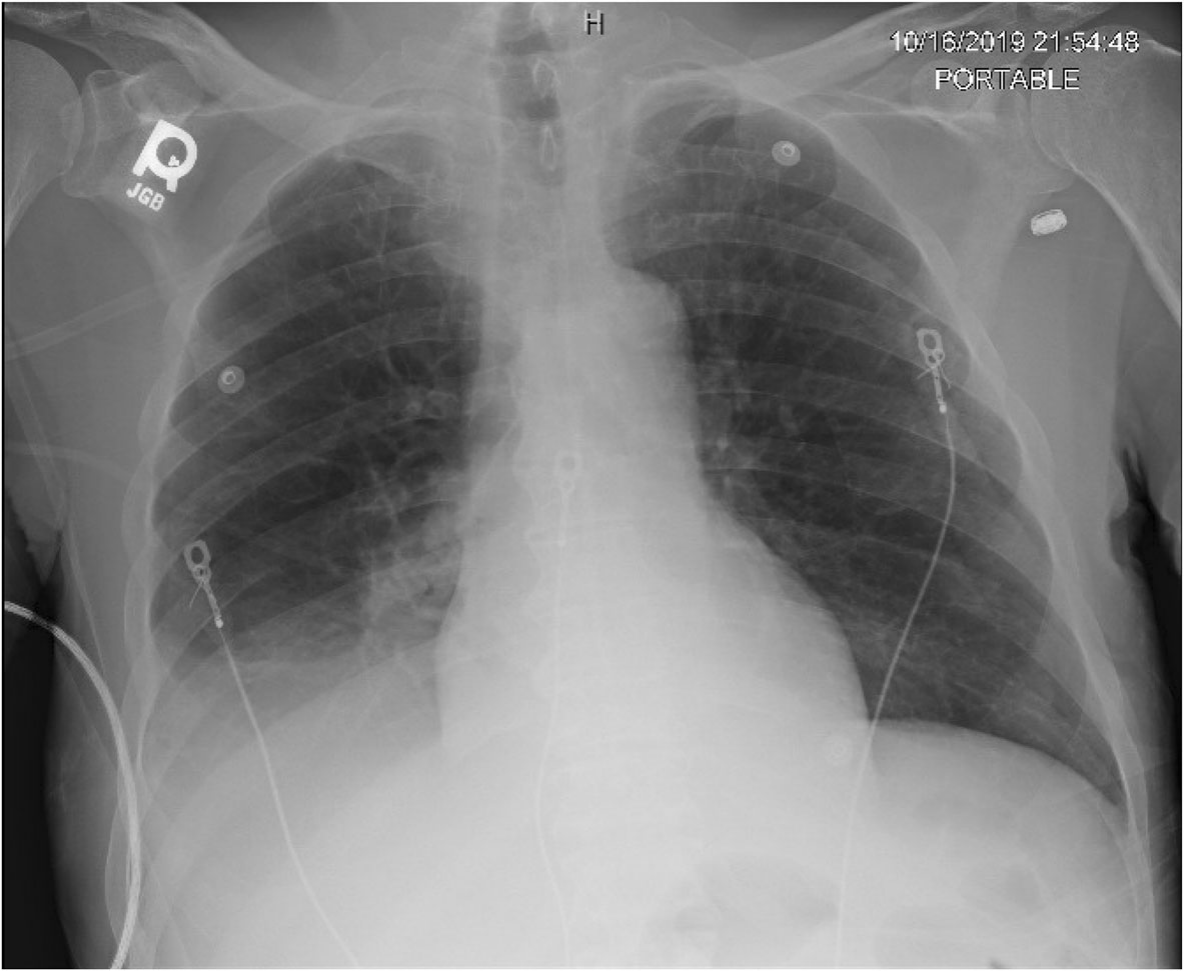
Anteroposterior chest X-ray showing a moderate right pleural effusion
